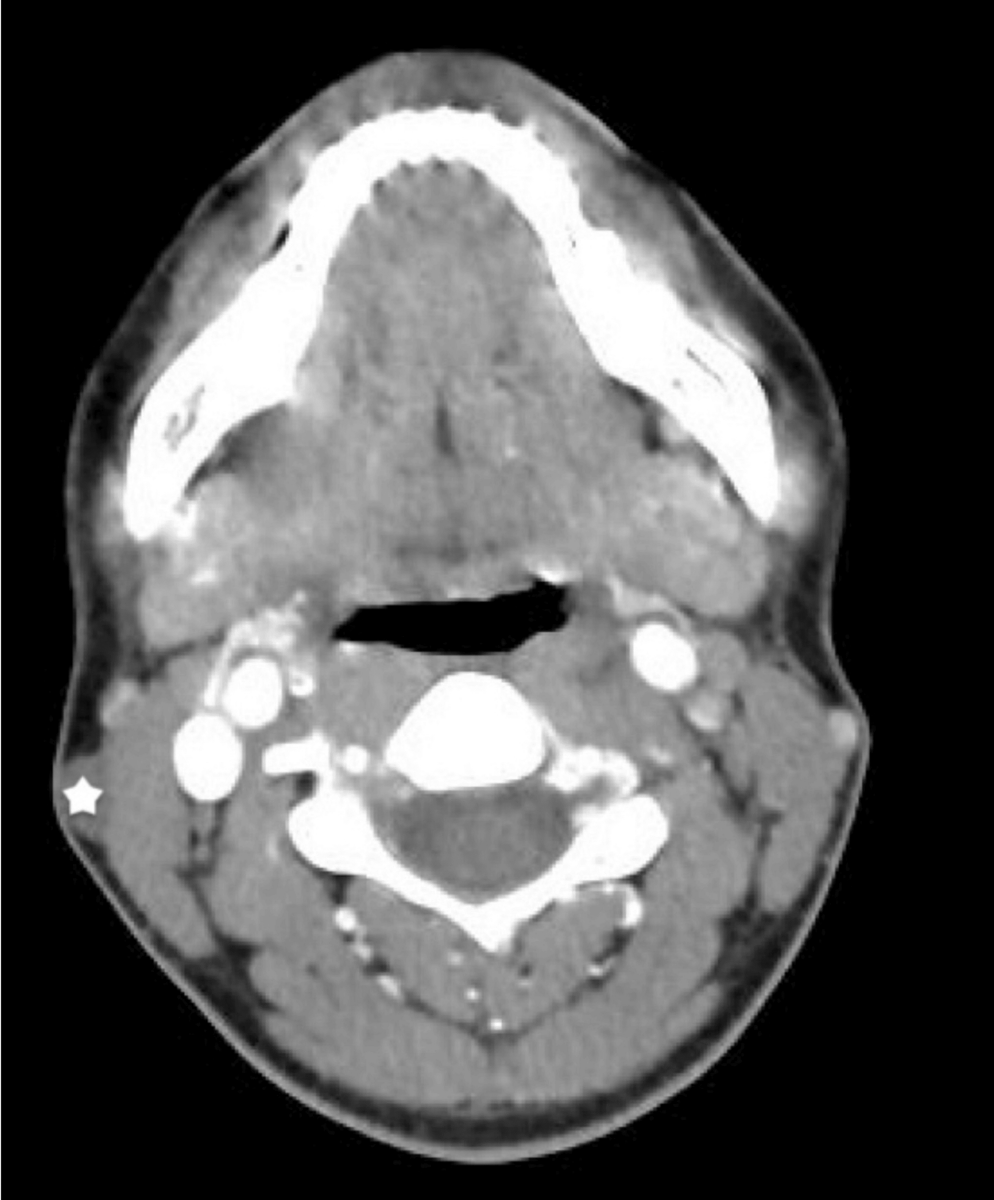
Contrast-enhanced CT scan of the neck showing an axial view of a neck mass, attached to the lateral aspect, middle third of the right sternocleidomastoid muscle (asterisk).
